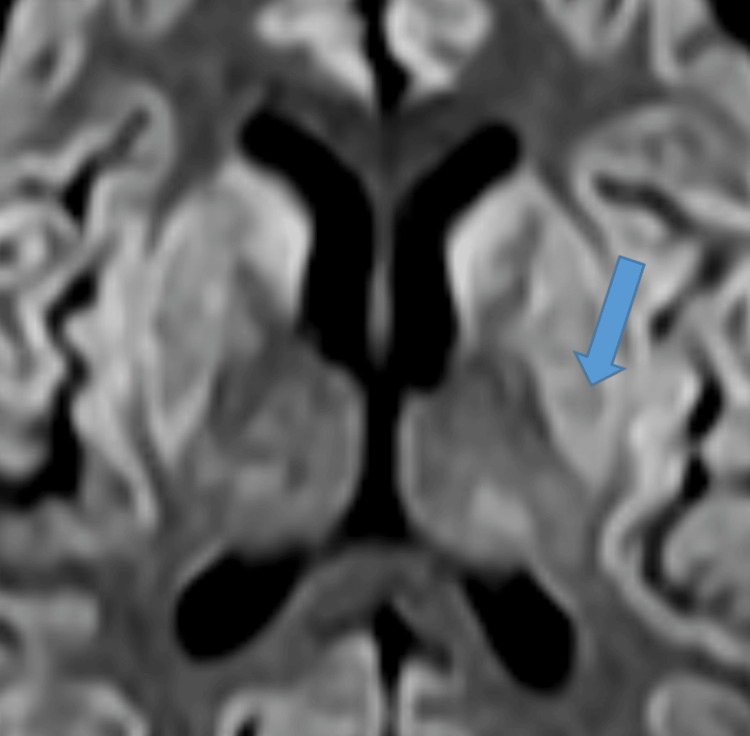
DWI Axial MRI Sequence Showing Hyperintense Signal (Blue Arrow). DWI: diffusion-weighted imaging; MRI: magnetic resonance imaging
